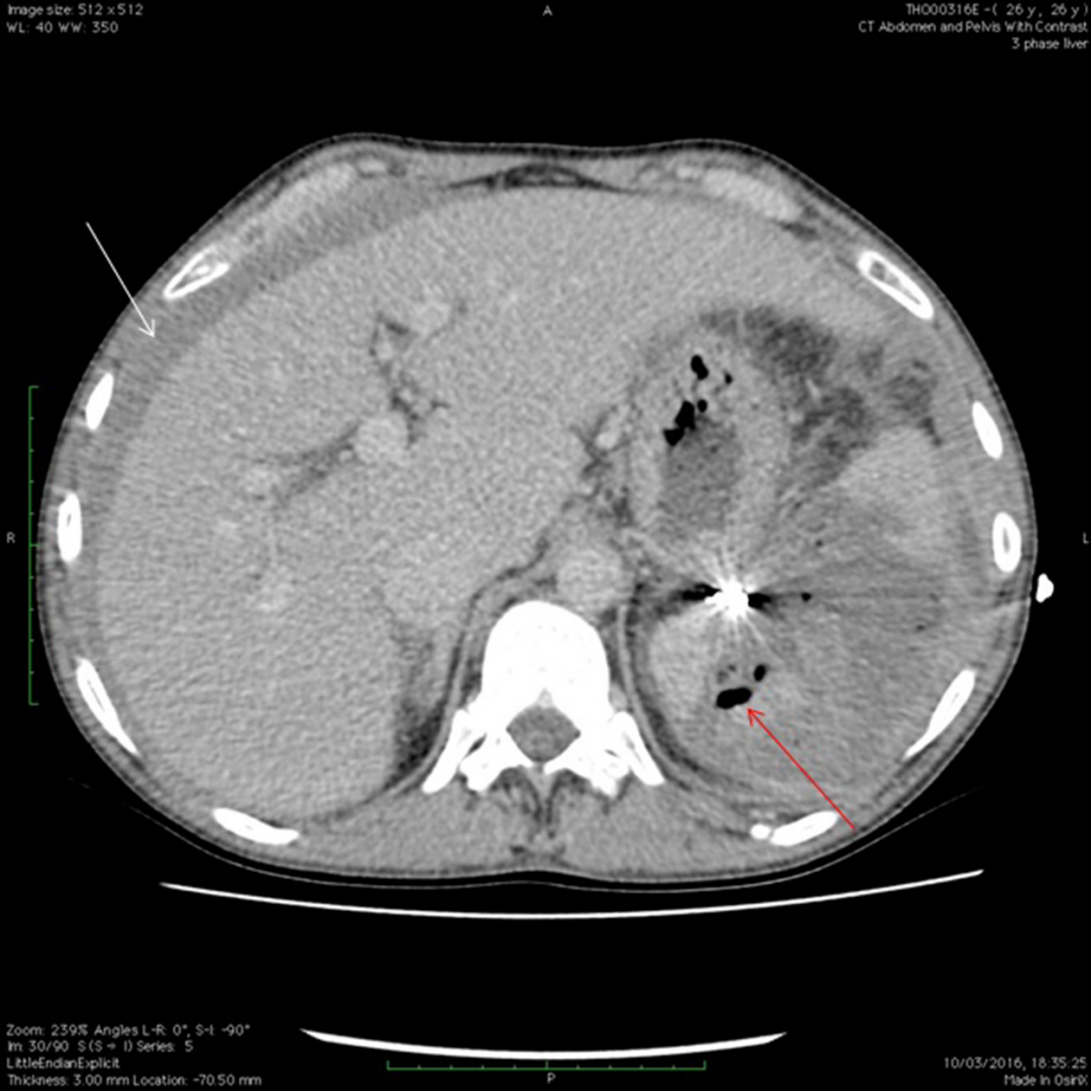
CT scan of abdomen post-embolisation therapy. Embolisation coils noted with a successful cessation of bleeding. Visible gas (red arrow) is seen in the spleen indicating infarction and necrosis. There is also a notable increase in extra-hepatic fluid (white arrow), that likely resulted from delayed presentation following the initial trauma.
